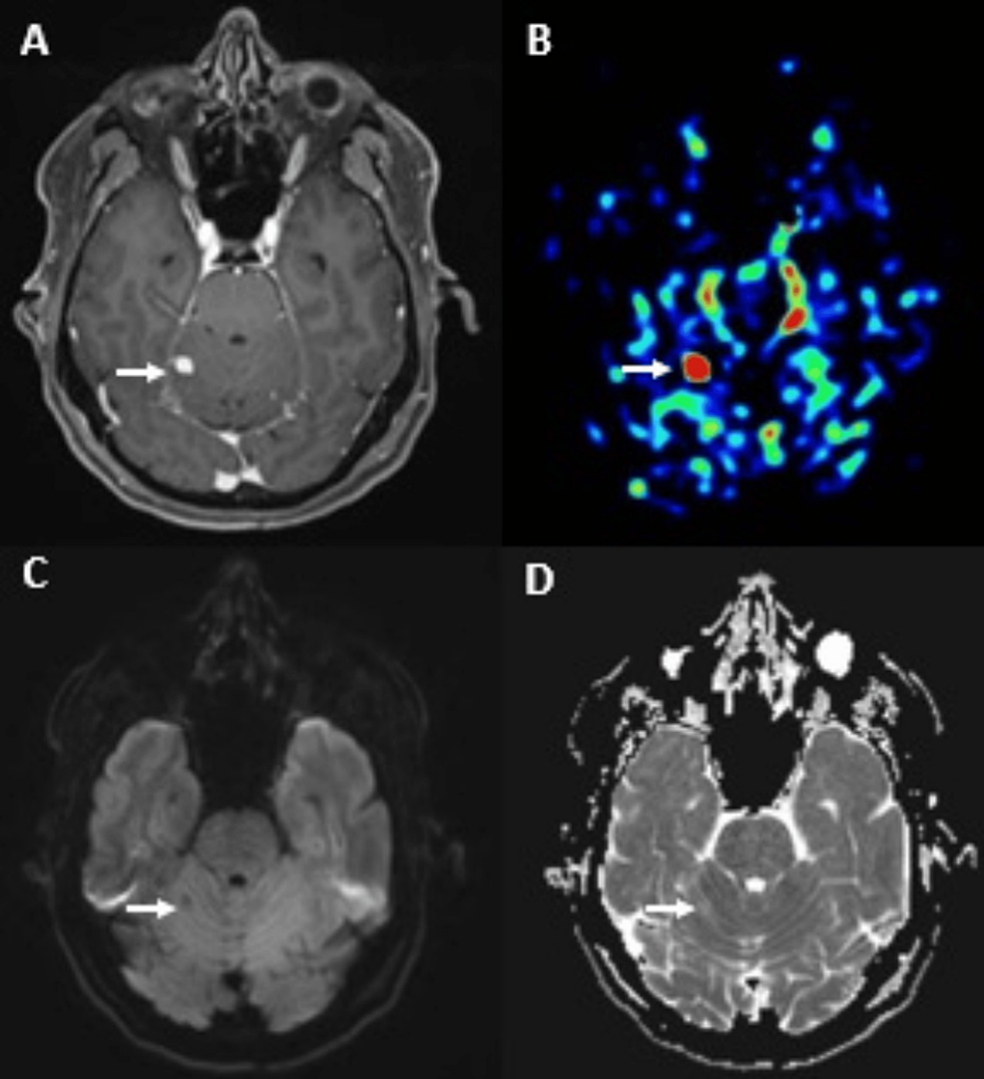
Brain magnetic resonance imaging (axial view). (A) Axial T1WI of brain MRI shows an intra-axial nodular lesion with homogeneous enhancement in the right cerebellar hemisphere (arrow) and increased cerebral blood flow (CBF) (B). Axial DWI shows a low signal (C) and a corresponding apparent diffusion coefficient (ADC) map (D) showing increased ADC of the nodule (arrow), which is very suggestive of cerebellar hemangioblastoma.
